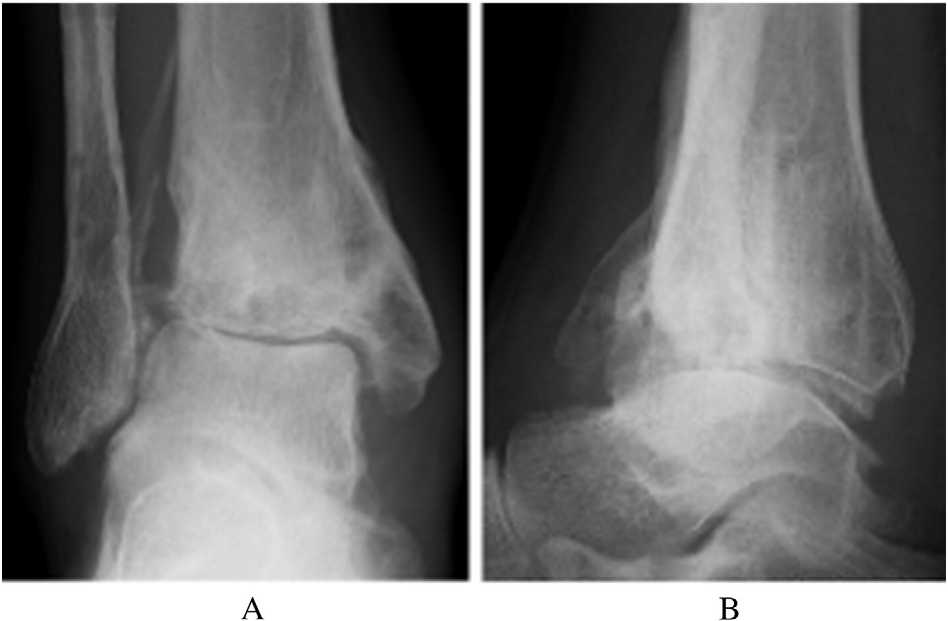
Radiographs at the initial examination showed an osteophyte in the anterolateral corner of the right plafond and moderate narrowing of the ankle joint. Forward displacement of the talus was seen: (A) anteroposterior view, (B) lateral view.
